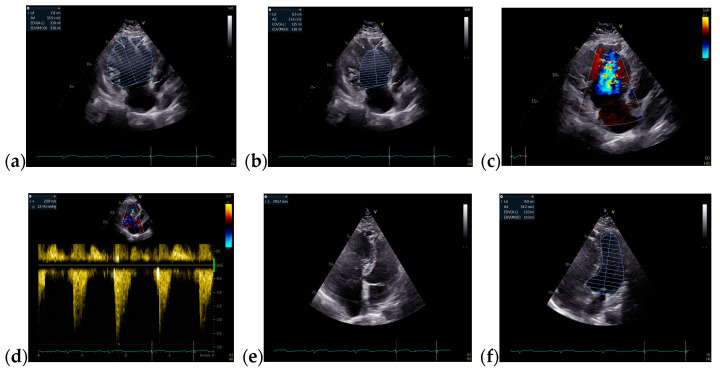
The TTE follow-up evaluation: (a) Apical 4-chamber view: dilated right cavities with a total volume of RV measuring 339 mL; (b) Apical 4-chamber view: atrialized RV volume of 135 mL; (c) Apical 4-chamber view: color Doppler flow revealing severe tricuspid regurgitation; (d) Tricuspid continuous wave Doppler flow: maximum RV-RA gradient = 33.45 mmHg; (e) Apical 4-chamber view: insertion of the septal cusp of the tricuspid valve at approximately 2.9 cm from the plane of the tricuspid annulus; (f) Apical 4-chamber view: end-diastolic left ventricular volume—110 mL. Abbreviations: TTE—transthoracic echocardiography; RV—right ventricle, RA—right atrium.
